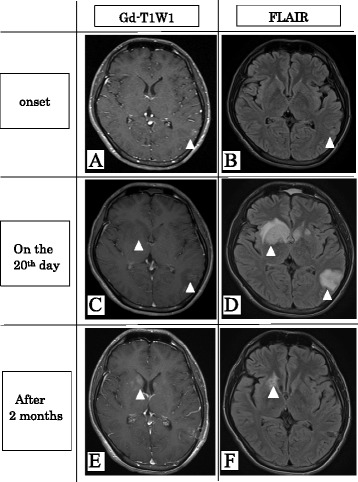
Axial T1-weighted MRI with gadolinium contrast (a) and FLAIR-weighted MRI (b) show mild enhancement and a high-intensity area, respectively, in the left temporal lobe at onset (*arrow heads*). Following periods of acute exacerbation on the 20th day, axial T1-weighted MRI with gadolinium contrast (c) showed slightly larger enhancement, and FLAIR-weighted MRI (d) revealed an asymmetrical hyperintense signal in the left temporal lobe and both basal nuclei. After 2 months of hospitalization, the abnormal lesions became smaller (e, f)
